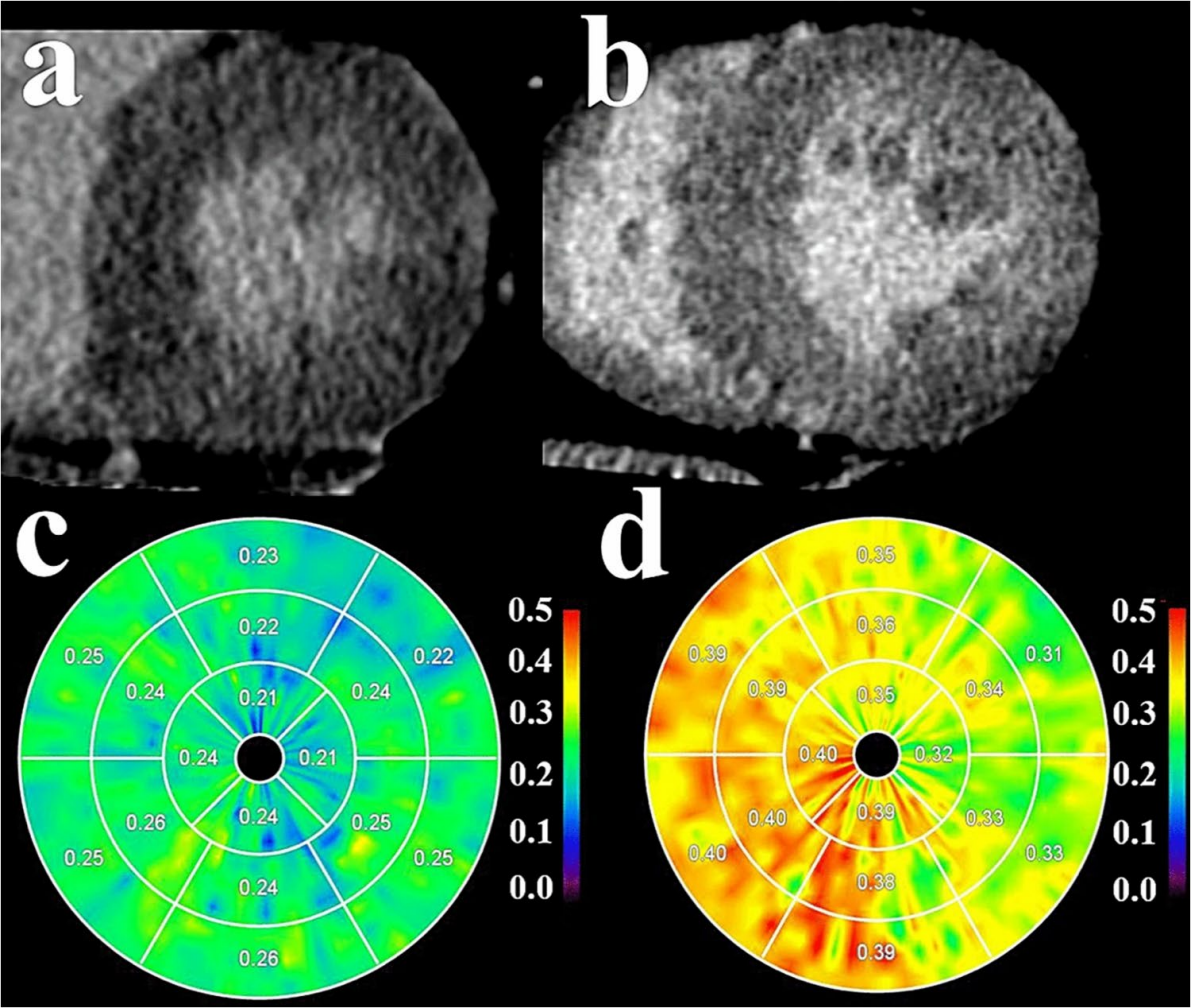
Calculation of ECV using CT. Delayed enhancement images and polar ECV maps of a patient with normal ECV (a and c) and a patient with increased ECV (b and d). ECV can be calculated by a subtraction image of a pre-contrast and a delayed enhancement post-contrast image. (By Yamada et al. [39] © CC-BY 4.0 https://creativecommons.org/licenses/by/4.0/.)
